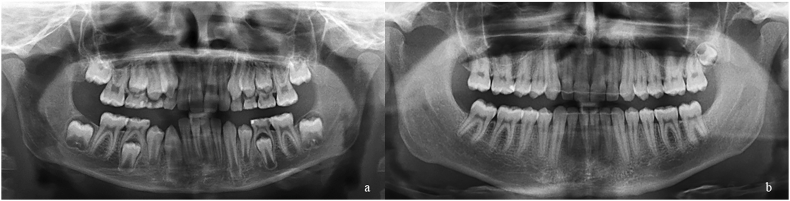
Panoramic radiographs before and after orthodontic treatment. a) pre-orthodontic treatment panoramic radiograph; b) post-orthodontic treatment panoramic radiograph showing first upper and lower molars presenting narrowing of the pulp space.
